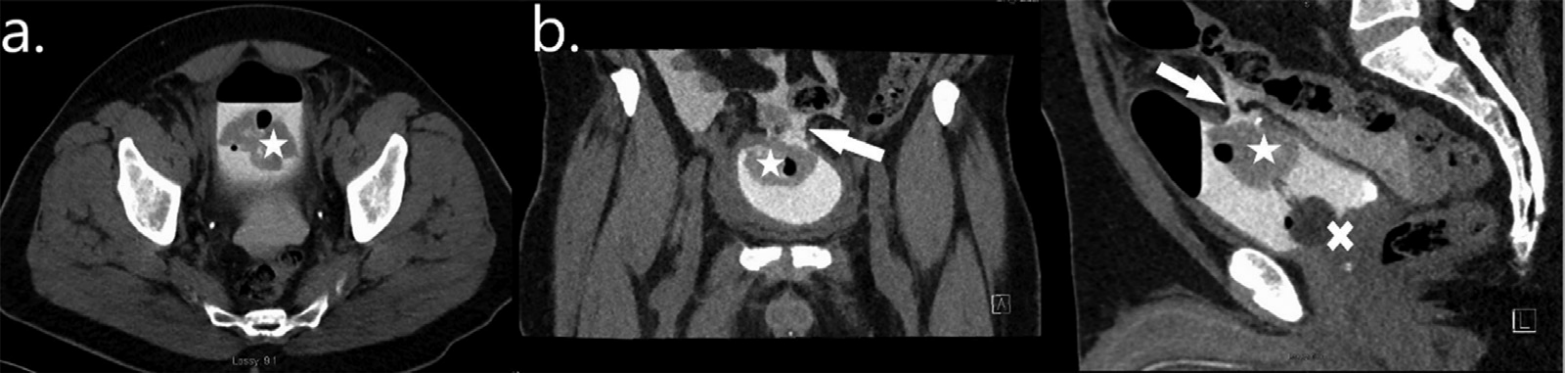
Case 4—Intraperitoneal bladder perforation following a minor fall: Axial (A), coronal (B), and sagittal (C) images from a CT cystogram demonstrating active contrast extravasation (arrows) with a large volume of clot (stars) within the bladder and a urethral catheter (cross) in situ.
